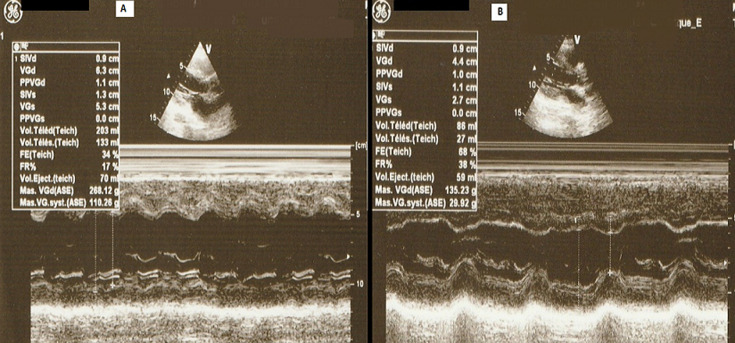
echocardiographic left ventricular M-mode images: (A) Day 1: LV diameter was enlarged, and the ejection fraction was decreased: (B) day 13: normal LVEF (60%) and wall thickness. LV = Left ventricle, LVE = left ventricle ejection fraction
